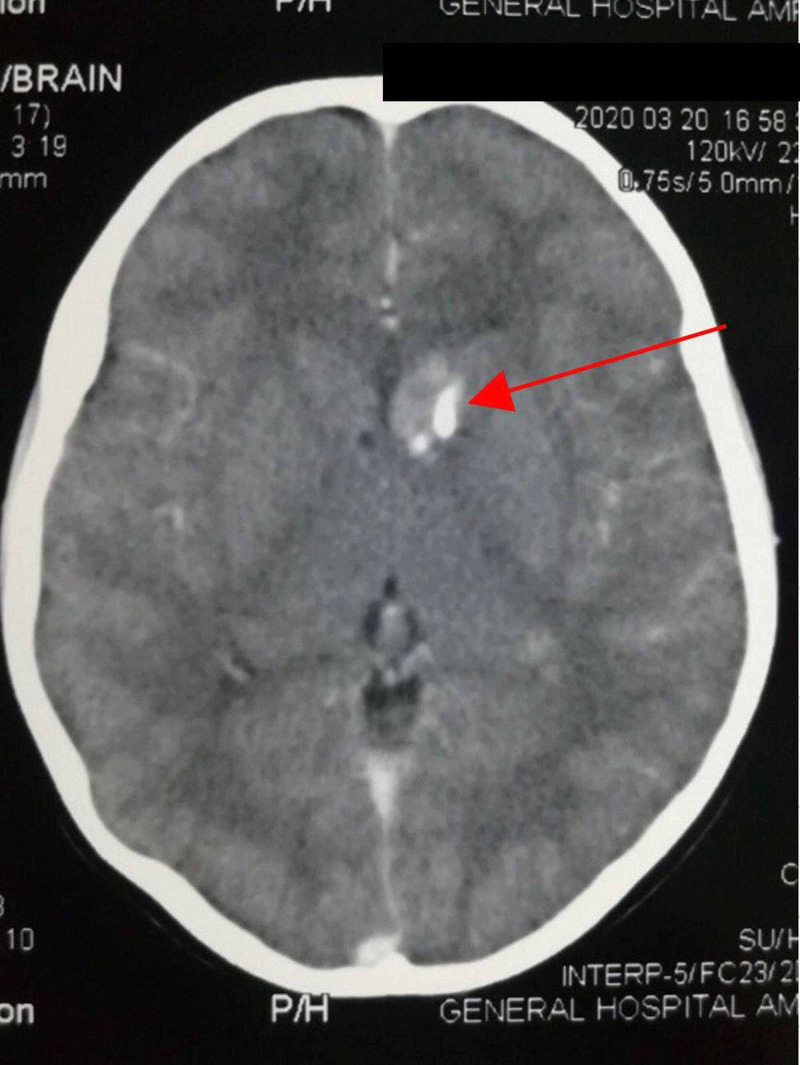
Contrast-enhanced CT showing 12 mm hyperdense mass adjacent to the left anterior horn of the lateral ventricle
